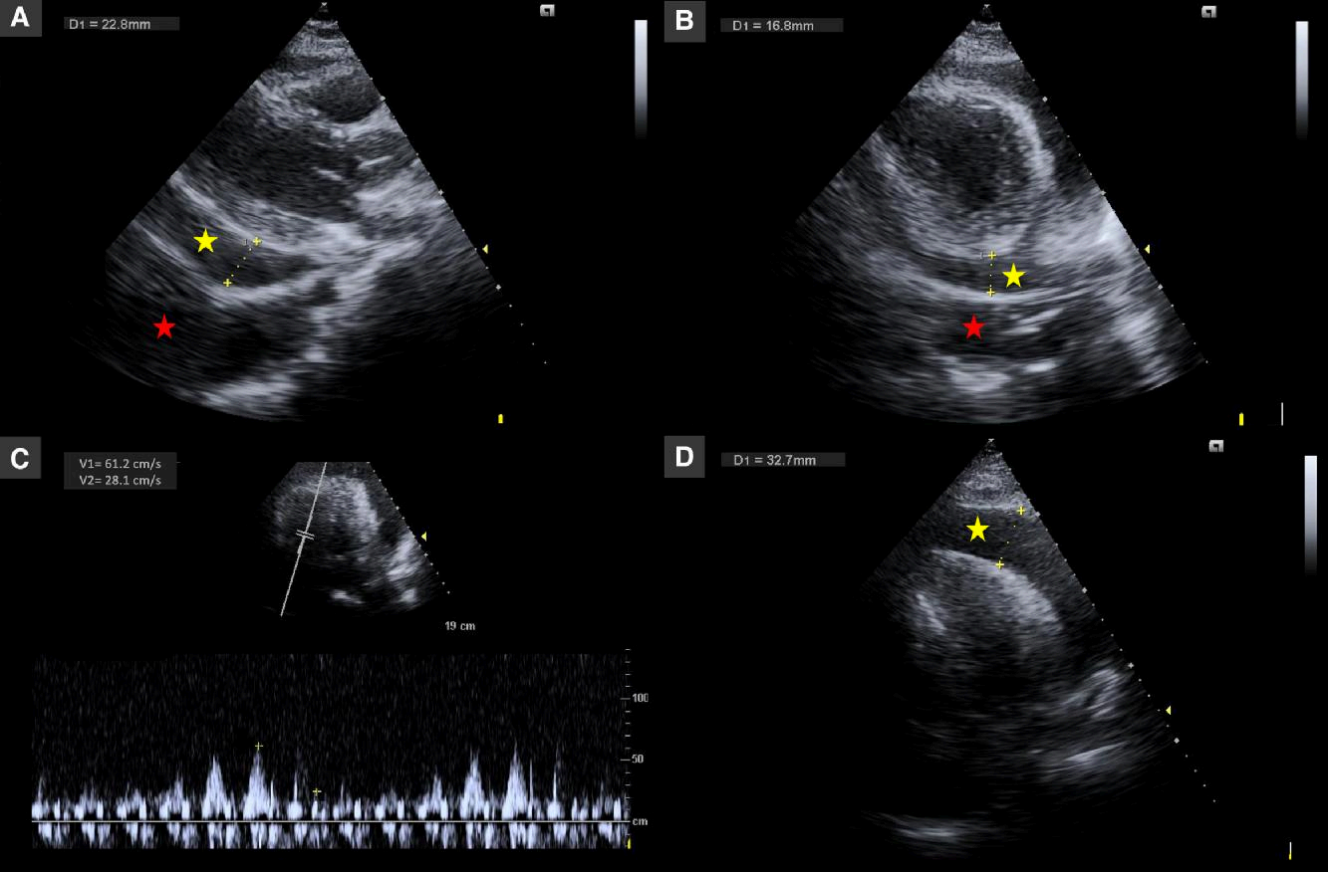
Echocardiography in parasternal long-axis (*A*), parasternal short-axis (*B*), and apical four-chamber (*C* and *D*) views showing pericardial effusion with large dimensions (30–33 mm, yellow star) with collapse of the right cavities and significant tricuspid inflow variation (46%) and pleural effusion (red star).
